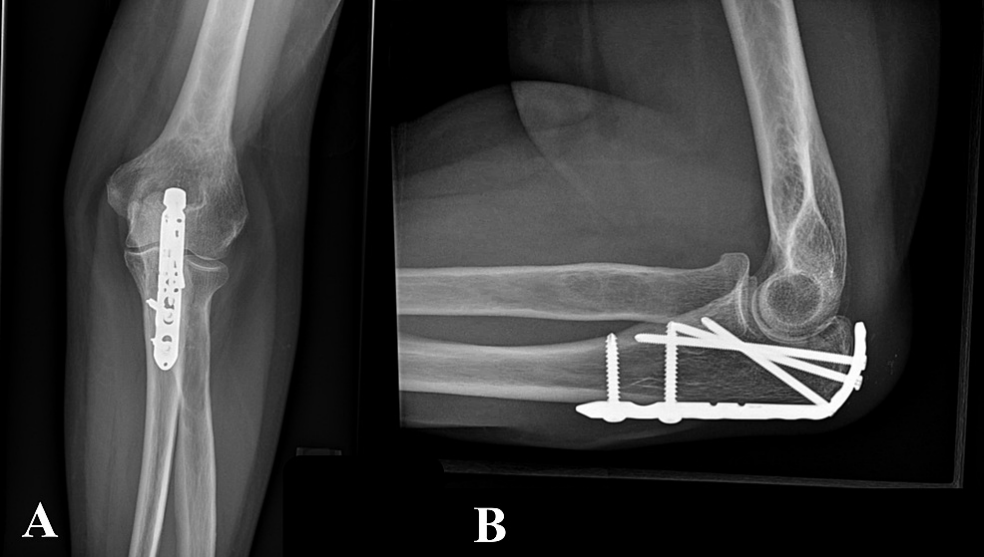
Follow-up radiographs of the left elbow at three months. Anteroposterior (A) and lateral radiographs (B) showing fracture healing in anatomic position and stable position of hardware.
